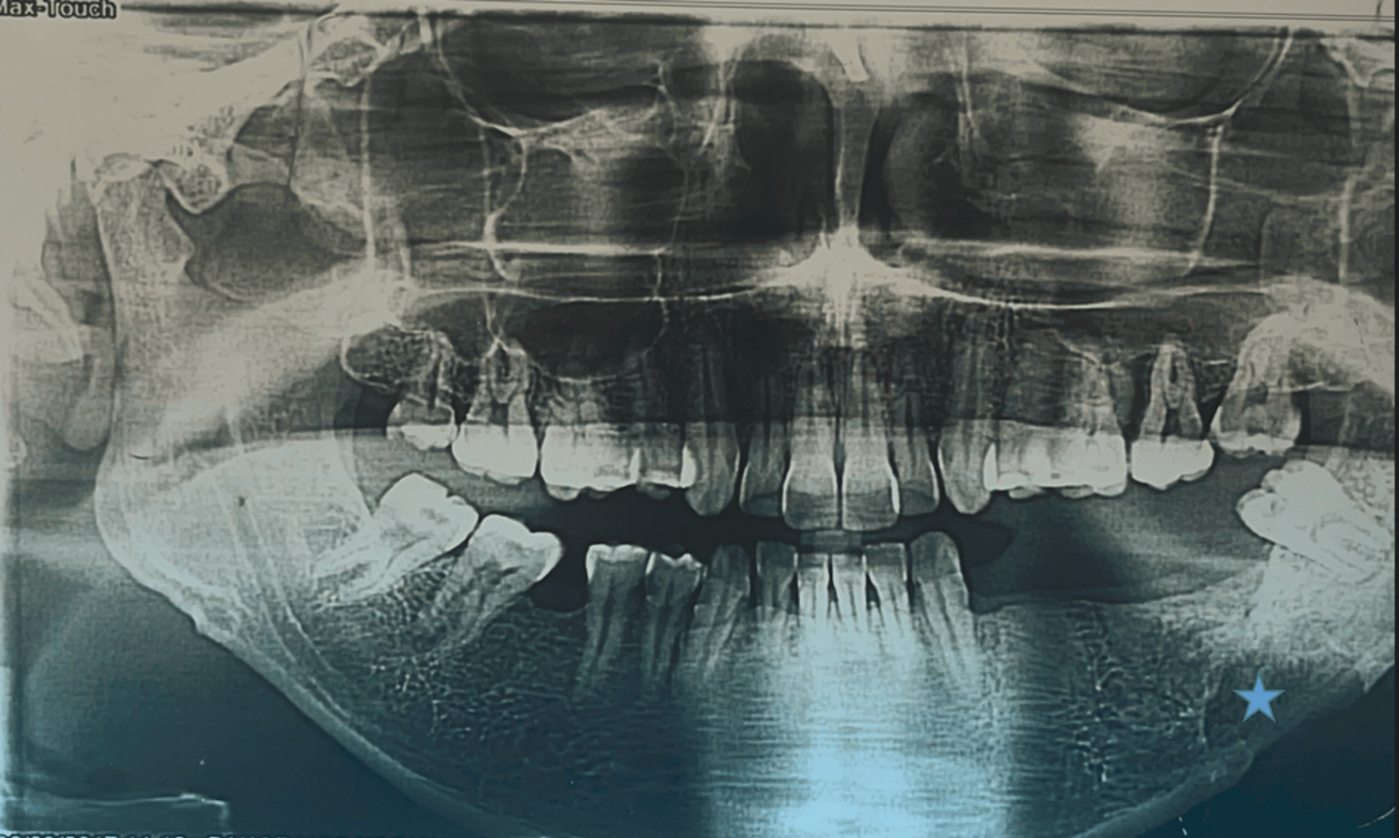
Panoramic X-ray: an irregular lacunar lesion (blue star) at the junction of the horizontal branch and the left mandibular angle, blowing out the cortical bone opposite.
